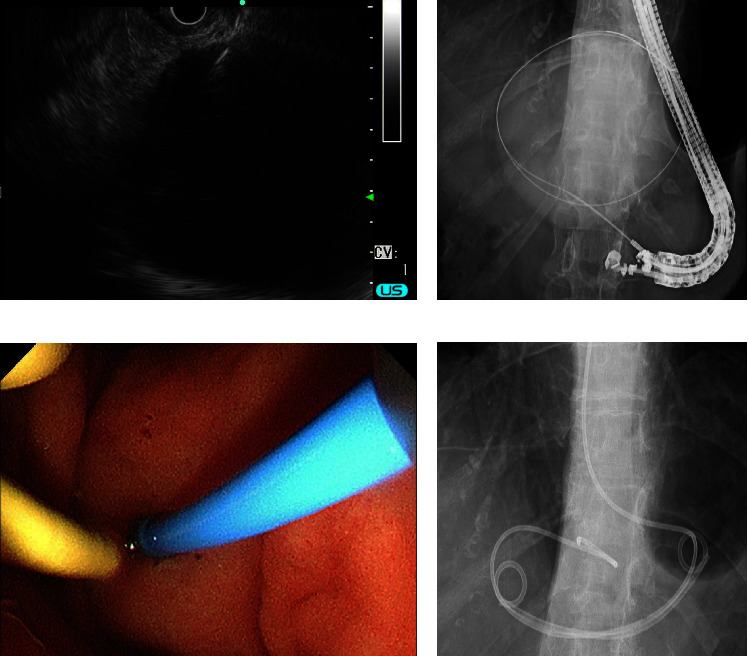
The liver cyst was visualized from the anterior wall of the gastric body using a therapeutic linear echoendoscope and punctured with a 19-gauge needle (a). Two guidewires were inserted into the liver cyst through a cannula (b). An internal 7-Fr loop diameter 10 cm double pigtail catheter and an external 6-Fr nasocystic drainage catheter were inserted into the liver cyst ((c) endoscopy; (d) X-ray).
